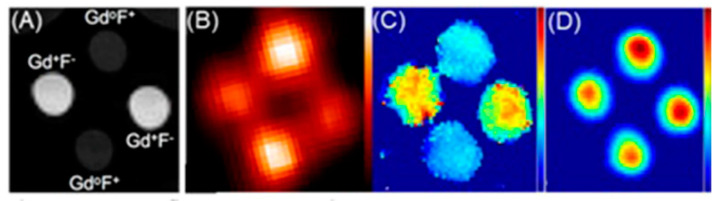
Imaging studies to detect hypoxia using *T*_1_-weighted MRI, PET, and EPRI. (A) *T*_1_-weighted MRI representing the presence and absence of Gd^III^-diethylenetriaminepentaacetic acid (defined in this example as Gd), and the presence or absence of ^18^F. The two variants of PET/EPRI imaging solution include: 1 mM of dFT, 1 mM of Gd and 25 µCi of 6 (defined as the Gd^+^F^−^ solution), and 1 mM of dFT and 49 µCi of 6 (defined as the Gd^o^F^+^ solution). (B) PET image intensity is related to the concentration of 6. (C) EPR image of Lorentzian line width, and (D) EPR image of dFT concentration. Image intensity is related to dFT concentration. Adapted with permission of IOP Publishing, Ltd., from A combined positron emission tomography (PET)-electron paramagnetic resonance imaging (EPRI) system: initial evaluation of a prototype scanner, Tseytlin, M.; Stolin, A. V.; Guggilapu, P.; Bobko, A. A.; Khramtsov, V. V.; Tseytlin, O.; Raylman, R. R. *63*, 2018; permission conveyed through Copyright Clearance Center, Inc. [287].
